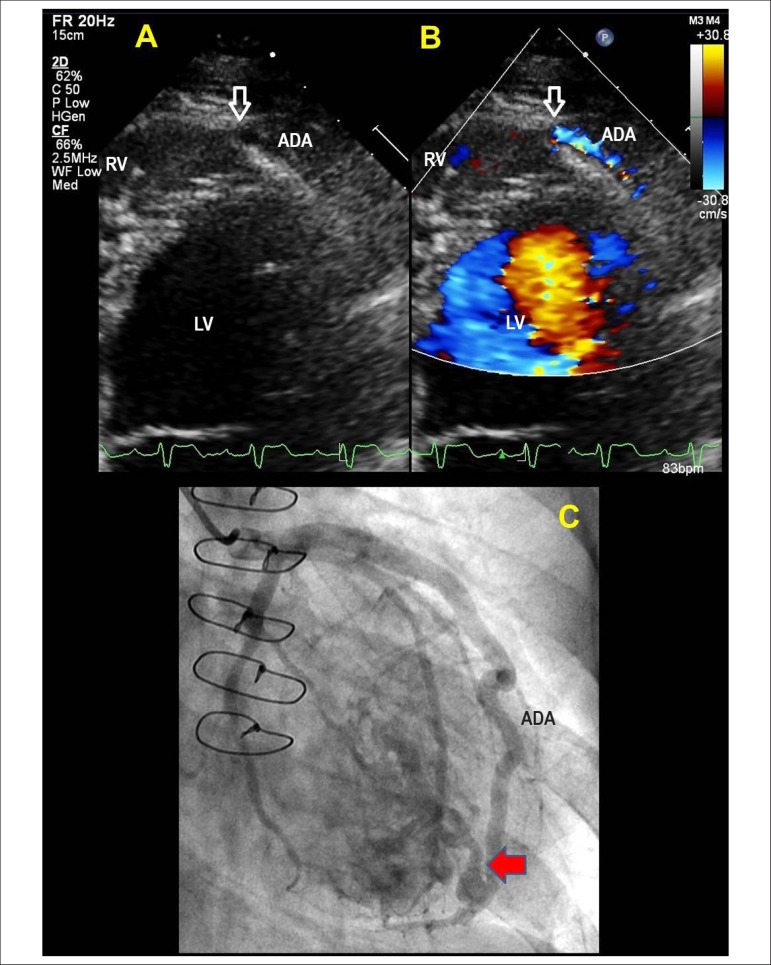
Images obtained from two-dimensional echocardiogram from the apical
